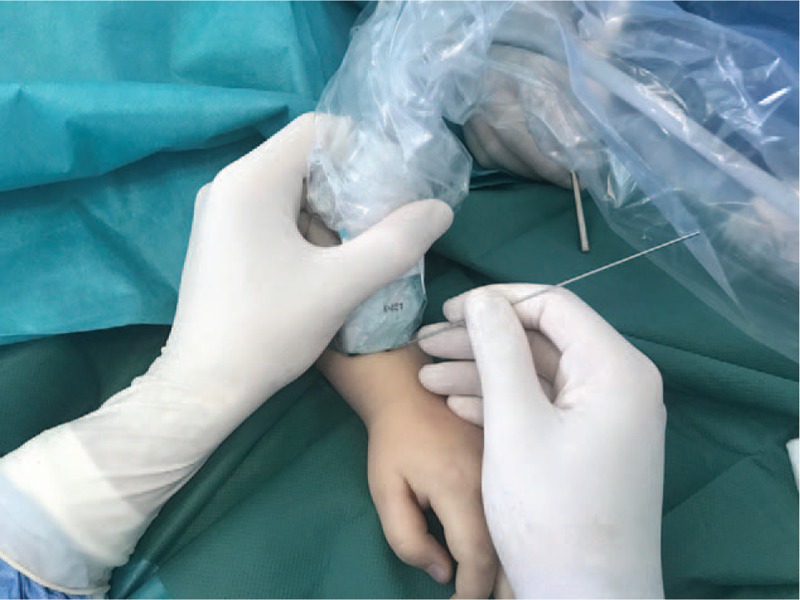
Sonography -assisted aiming of the radial side of Lister's tubecule with a K-wire.
